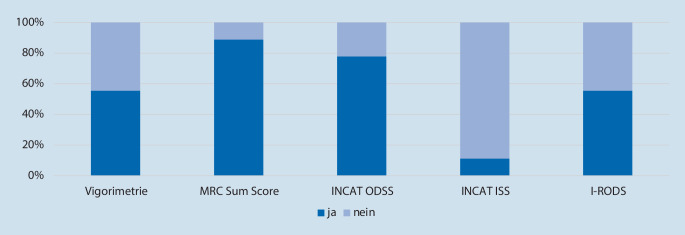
Die zeitlichen Abstände zwischen elektrophysiologischen und nervensonografischen Verlaufskontrollen bzw. der Umfang solcher Kontrollen wurden als sehr variabel berichtet.
